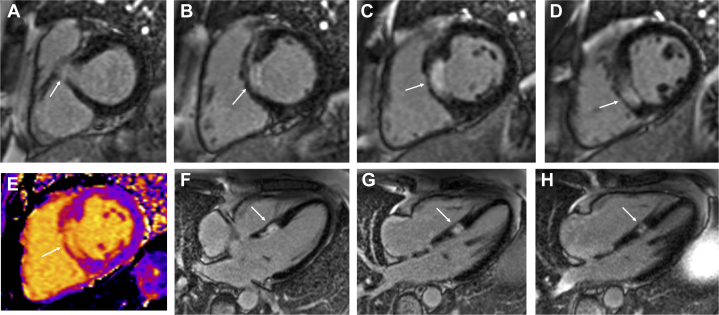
Cardiac Magnetic Resonance Imaging Cardiac magnetic resonance short-axis late gadolinium enhancement images (A to D), short-axis T1 map (E), and 4-chamber late gadolinium enhancement images (F to H) demonstrating transmural infarction of the septum at basal and mid-ventricular levels. There is no evidence of microvascular obstruction, myocardial edema, or pericardial effusion. Arrows indicate the infarct location.
